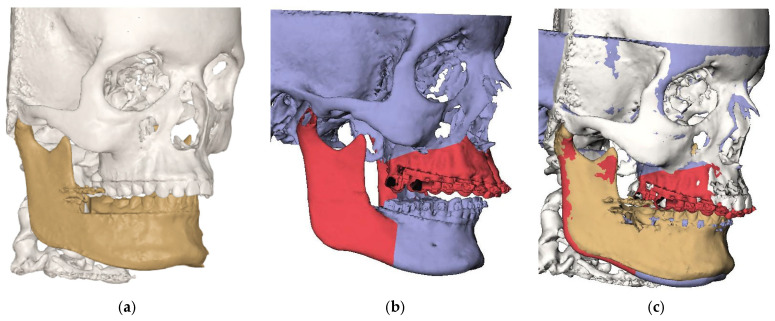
Registration of CBCT T0 towards surgery planning. (a) CBCT T0; (b) virtual head model T1; (c) registration of T0 model towards T1 model.
